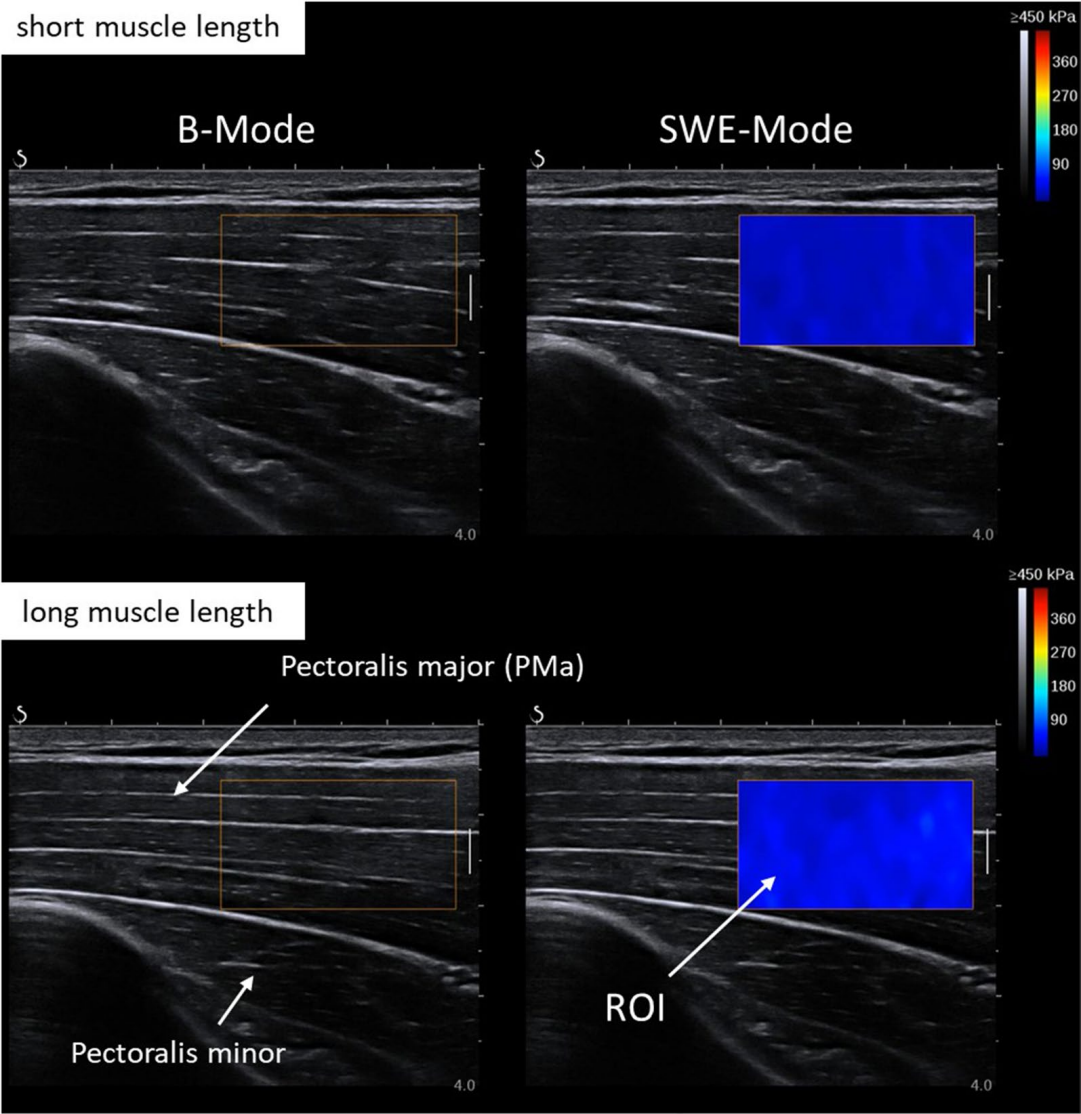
Example of an ultrasound of the pectoralis major (PMa) muscle (pars clavicularis) at a long (top) and short muscle length (bottom), in a relaxed state. The lighter blue in the ROI in the lower picture signalizes a higher muscle stiffness, as it occurs in the longer muscle length. SWE, shear wave elastography; PMa, pectoralis major; ROI, region of interest
